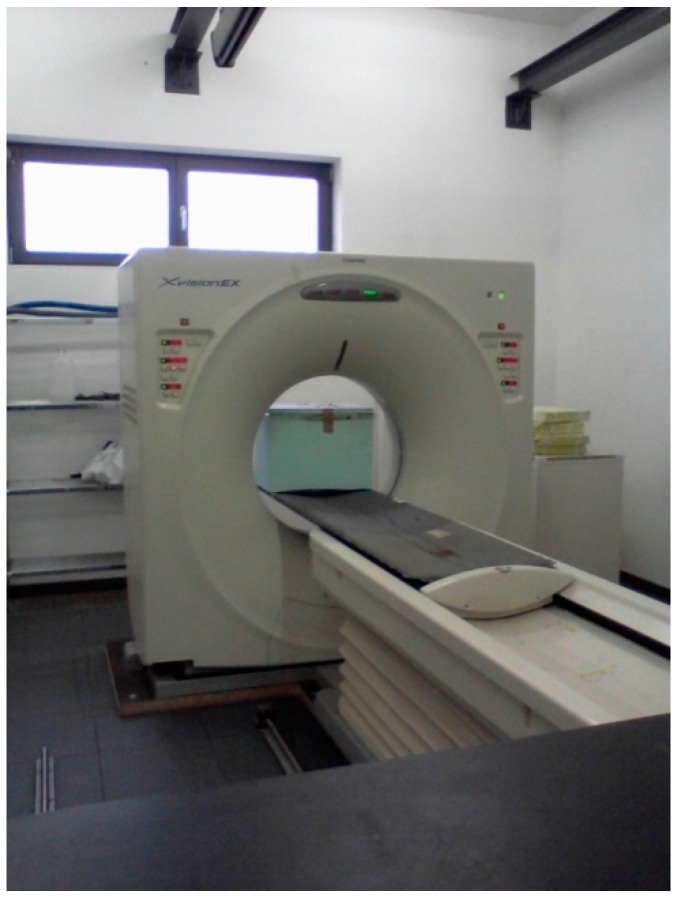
Bee colony placed in a macro-computed tomography scanner (macroCT).
